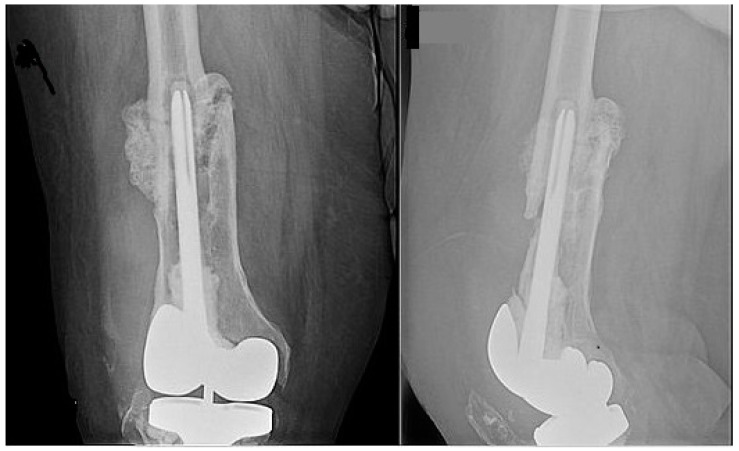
Xray anterior-posterior and lateral views of the right femur showing prior incompletely united distal femoral shaft fracture.
